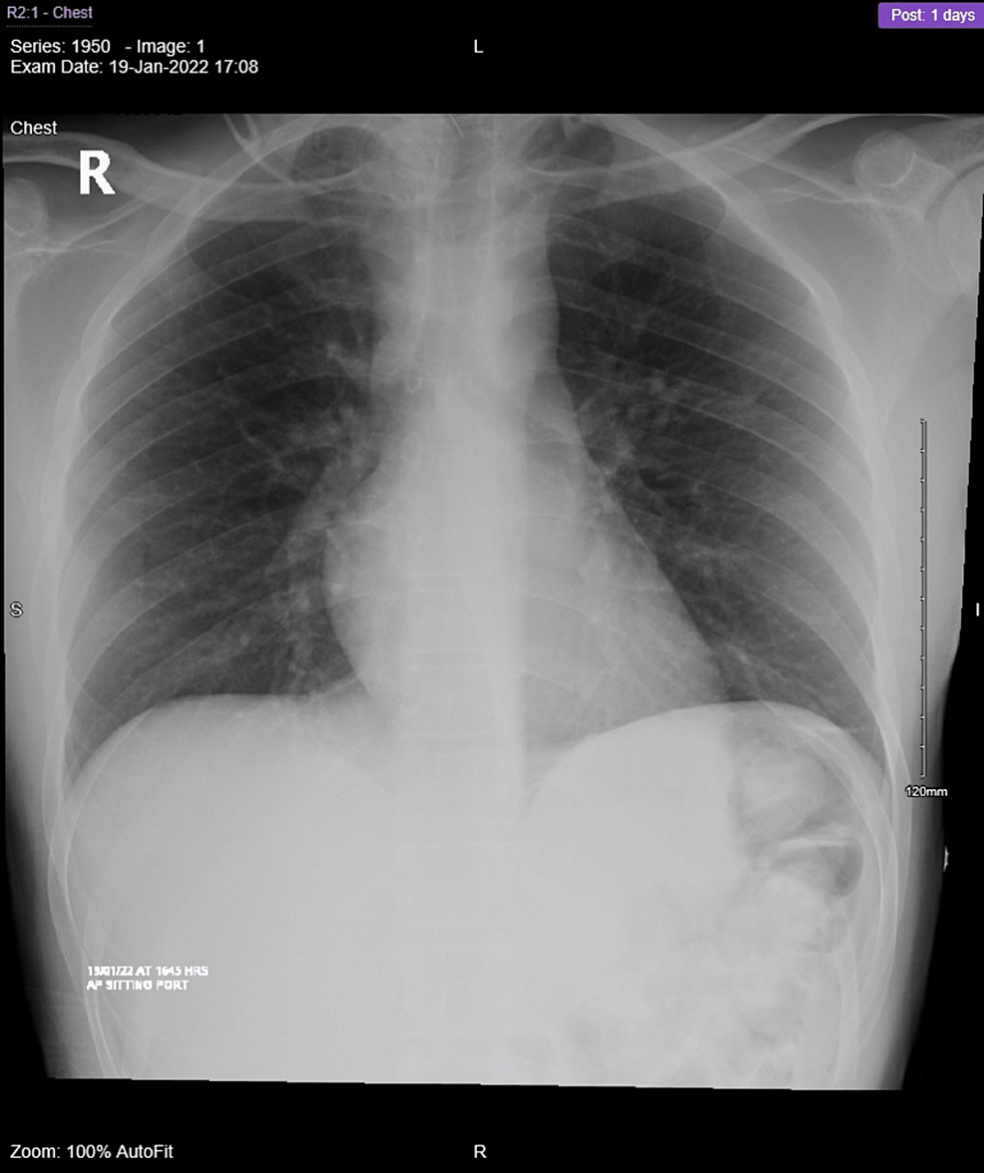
Chest X-ray of the patient. Done during the assessment pre-operative period. It shows no consolidation, pleural effusion, or pneumothorax.
